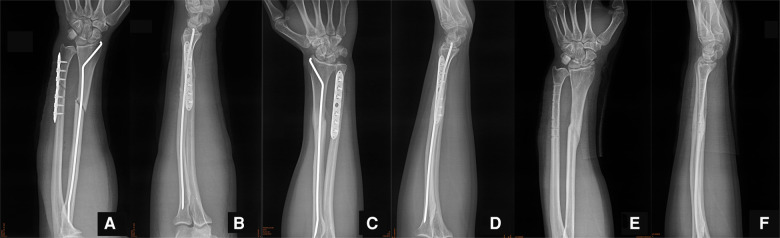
(A,B) Postoperative x-ray films of the left forearm. (C,D) X-ray films of the patient at 1 month after surgery. (E,F) X-ray films of the patient after removal of the internal fixation at 12 months after surgery.
